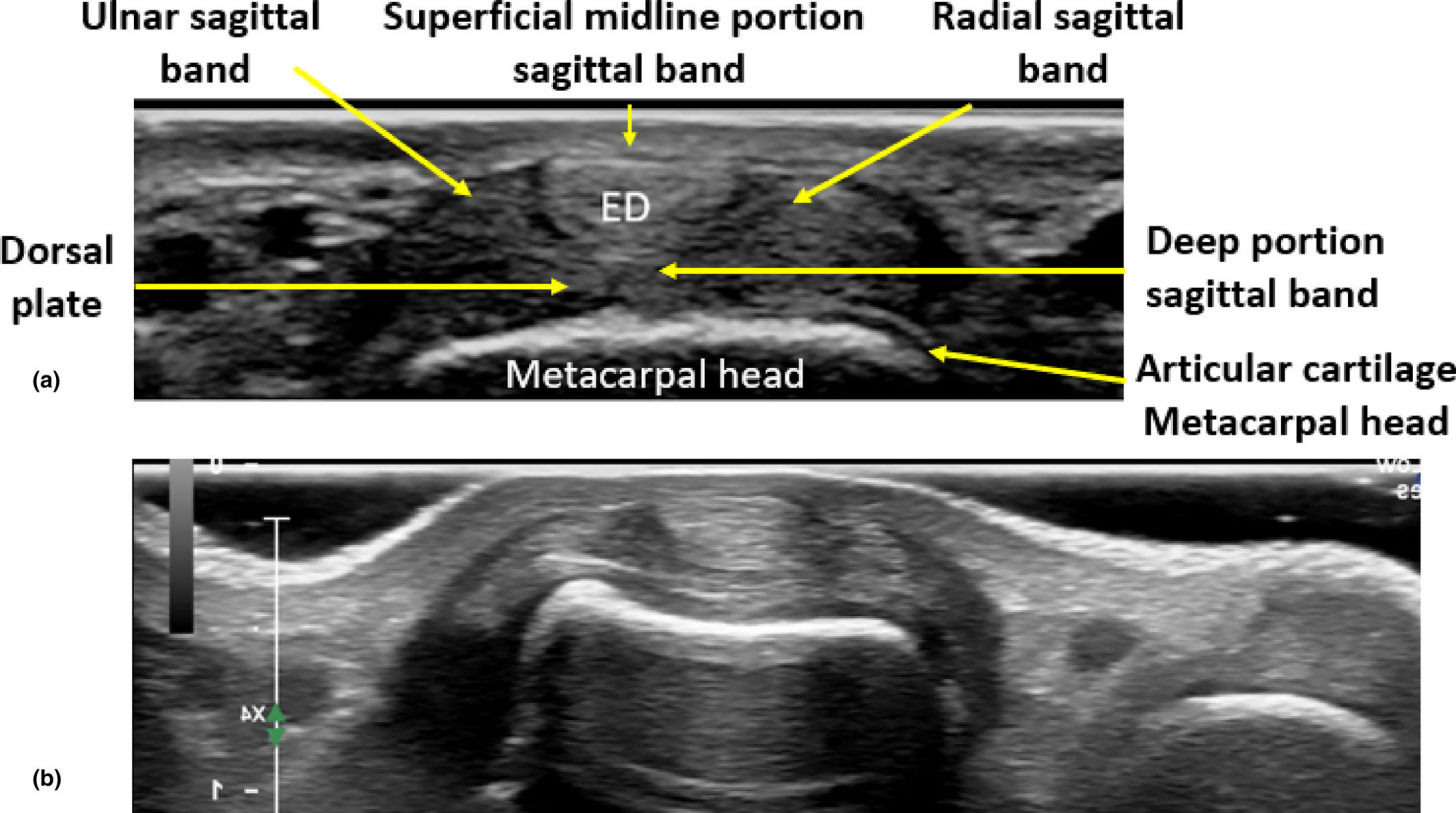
Short‐axis sonographic image of the dorsal aspect of the uninjured third MCPJ with (a) and without (b) labels. The ED tendon is demonstrated in short axis. The superficial and deep portions of the sagittal band in midline are seen with the deep portion superficial to the dorsal plate and the articular cartilage of the metacarpal head. The radial and ulnar portions of the sagittal band are demonstrated. ED, extensor digitorum; MCPJ, metacarpophalyngeal joint.
